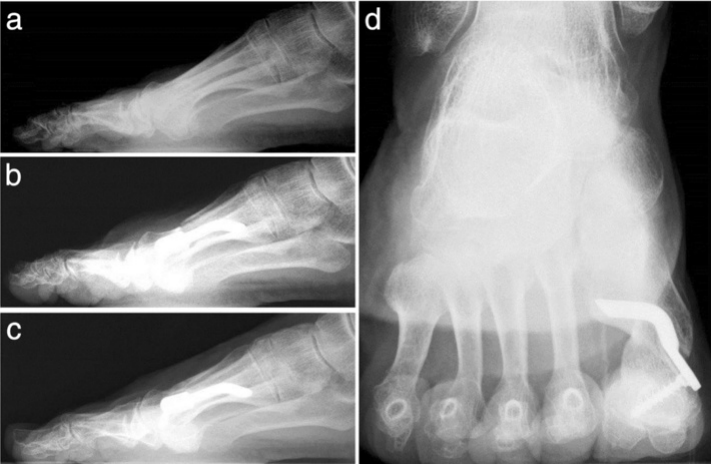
Case 12. A 71-year-old woman with moderate HV. a Preoperative lateral radiographic image. b At 1-month follow-up, lateral X-ray image. c Radiographic aspect at 48-month follow-up showing the maintained correction of the deformity and complete healing of the osteotomy. d X-ray image, sesamoid axial view, at 48-month follow-up
